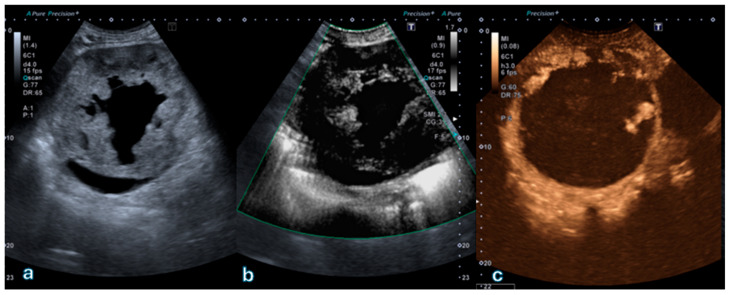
Partially cystic renal lesion with an eccentrically located, heterogeneously hyperechoic solid component. (a) B-mode ultrasound shows a mixed cystic–solid lesion with irregular internal architecture. (b) Superb Microvascular Imaging (SMI) demonstrates distinct microvascular flow signals within the solid component, indicating true vascularity. (c) Corresponding contrast-enhanced ultrasound (CEUS) image confirms contrast enhancement of the same solid area, consistent with a Bosniak IV lesion according to the 2019 Bosniak classification. This case exemplifies complete concordance between SMI and CEUS in detecting vascularized mural components suggestive of malignancy.
